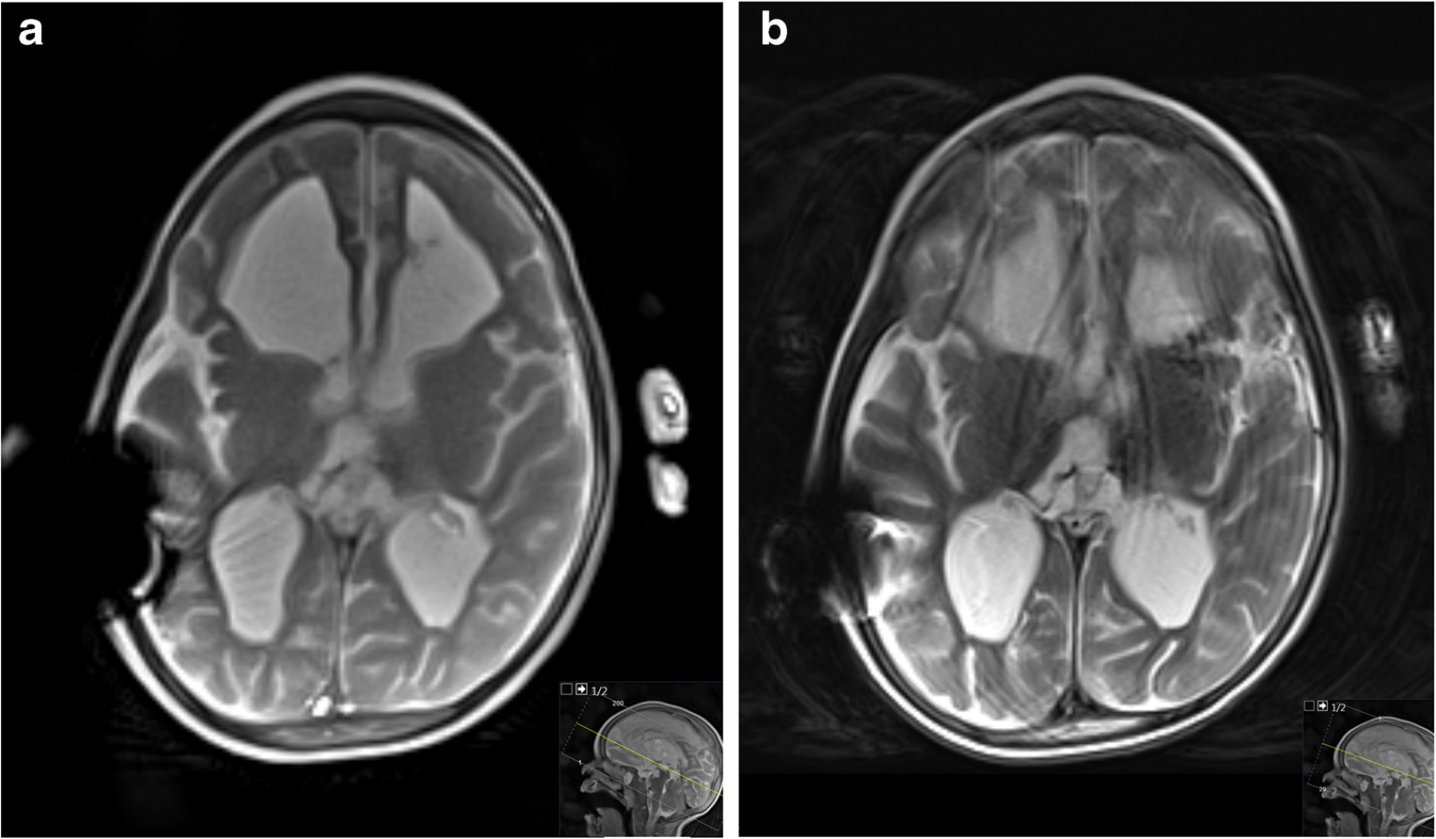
Intracranial bleeding and a ventriculoperitoneal shunt causing local susceptibility-induced signal void in a 2.3-year-old boy. a, b Comparison of (a) a transverse T2/T1-weighted volume coverage scan (11 s) based on cross-sectional real-time MRI without motion artifacts and (b) a conventional T2-weighted fast spin-echo (FSE) sequence (38 s) with motion artifacts
